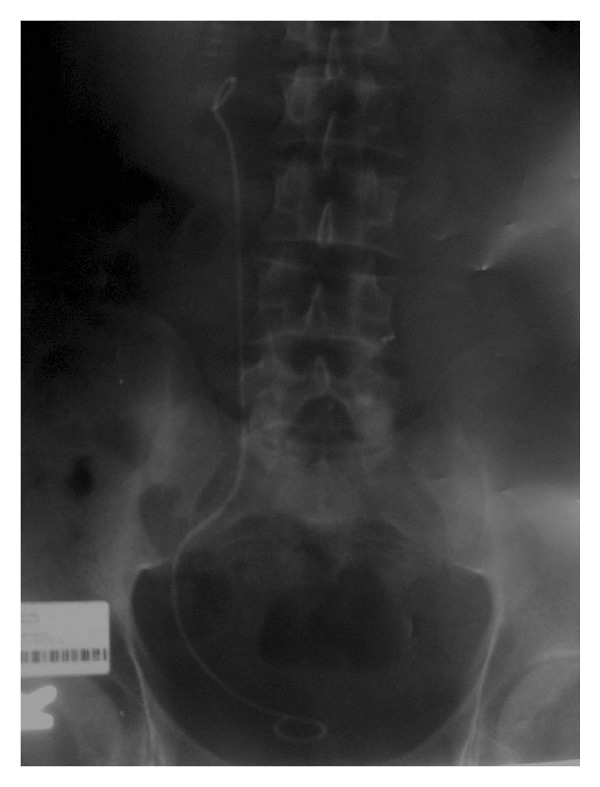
Kidney, ureter, and bladder (KUB) X-ray showing proper stent placement.
